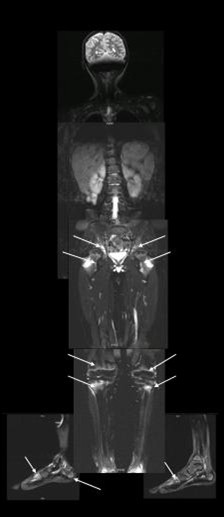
Whole-body magnetic resonance imaging of chronic nonbacterial osteomyelitis. Whole-body magnetic resonance imaging of one patient with extensive multifocal inflammatory radiological lesions at time of diagnosis: T2-weighted images with fat suppression (inverse recovery sequences, TIRM). The os sacrum and the acetabulum (all three osseous parts) did show severe signal elevation in the TIRM sequence. Further lesions are seen in the metaphyses of both proximal and distal femurs, proximal tibias and fibulas predominantly in the epiphyses/metaphyses and in the distal tibia and fibula with periosteal edema on the right side, supporting the clinical diagnosis of periostitis and arthritis. Signal alterations/edema in the skeleton of the feet can be noted at the basis of os metatarsale V, os metatarsale I and in the tuber calcanei on the right side; on the left side, the basis and the proximal parts of os metatarsale I and the distal os metatarsale V and the proximal phalanx V are affected.
